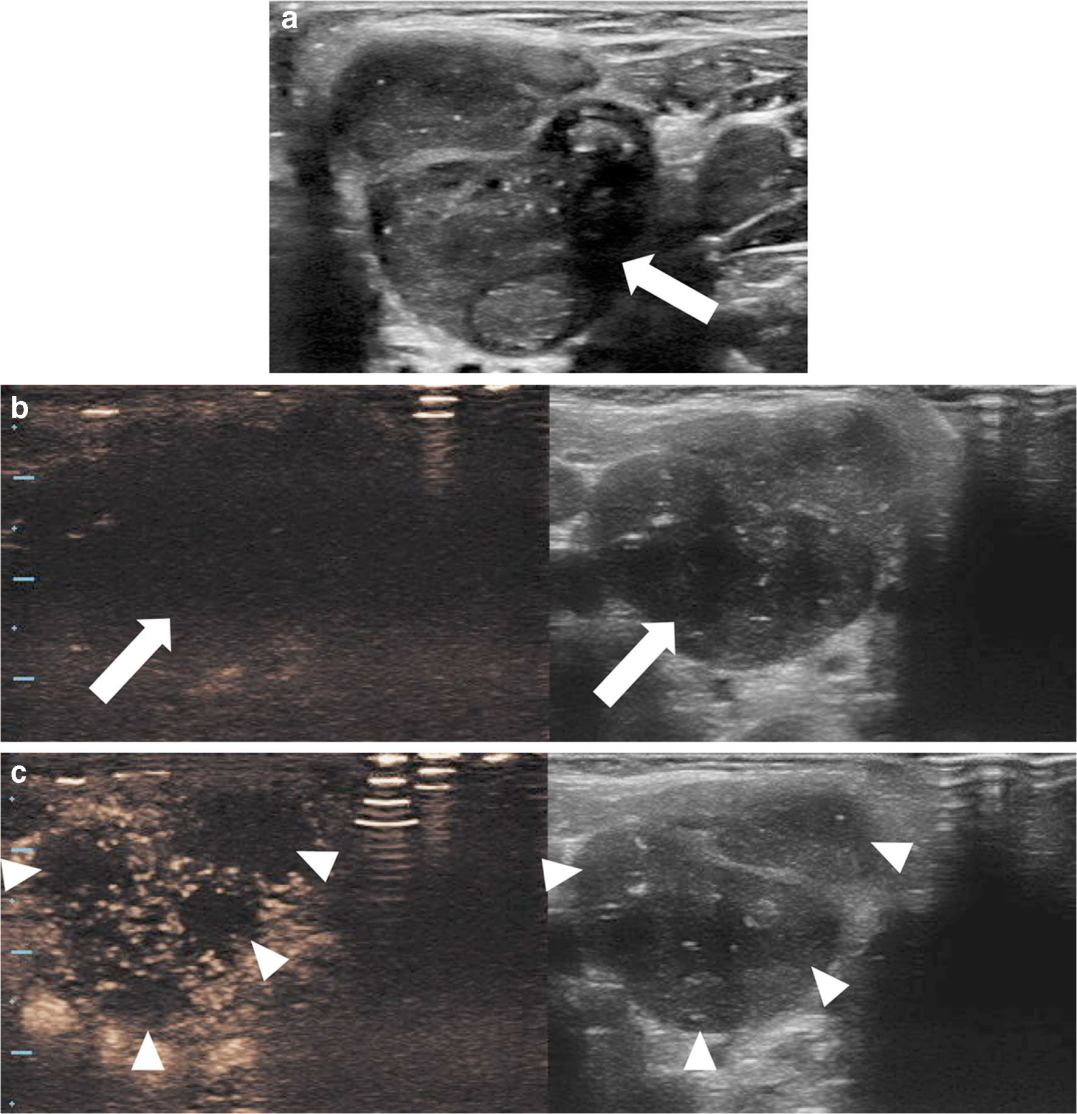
Atypical cervical mycobacteriosis in a 3-year-old girl. a Transverse gray-scale US demonstrates an enlarged lymph node (*arrow*) with heterogeneous internal reflectivity and presence of tiny hyperechoic punctate foci. b, c Transverse contrast-enhanced ultrasound (CEUS) with dual display of contrast (*left*) and gray-scale (*right*) modes. Ten seconds post contrast injection (b), no enhancement is seen within the lymph node (*arrow*). Fifteen seconds post contrast injection (c), there are multiple areas (*arrowheads*) of nonenhancement centrally within the lymph node, corresponding to caseating necrosis suggestive of atypical mycobacteriosis within the lymph node
